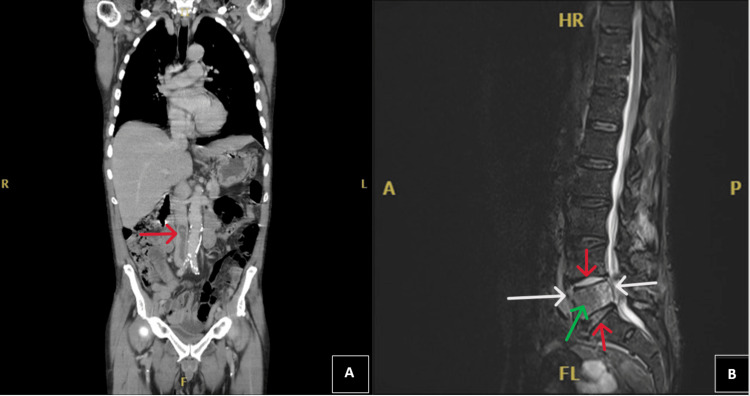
CT and MRI of the patient (A) CT of the thorax, abdomen, and pelvis. Coronal view showing an air-containing thrombus extending from the left common iliac vein to the IVC, marked with a red arrow. (B) MRI of the whole spine. Sagittal view showing emphysematous osteomyelitis of the L5 vertebral body (green arrow) and L4/L5 and L5/S1 discitis (red arrows). A prevertebral collection and epidural collection (white arrow) within the spinal canal are also seen at the same level CT: computed tomography; MRI: magnetic resonance imaging; IVC: inferior vena cava
